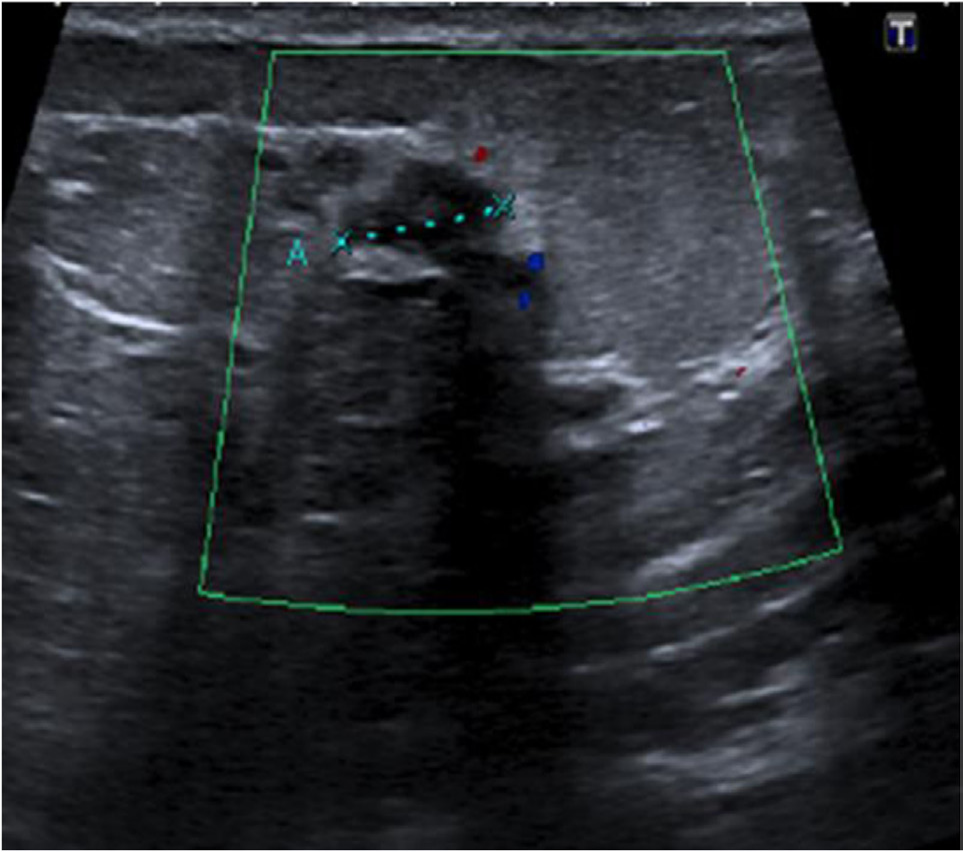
Ultrasound right nipple: suspect galactophoric dilatation of the right supero-external quadrant.
